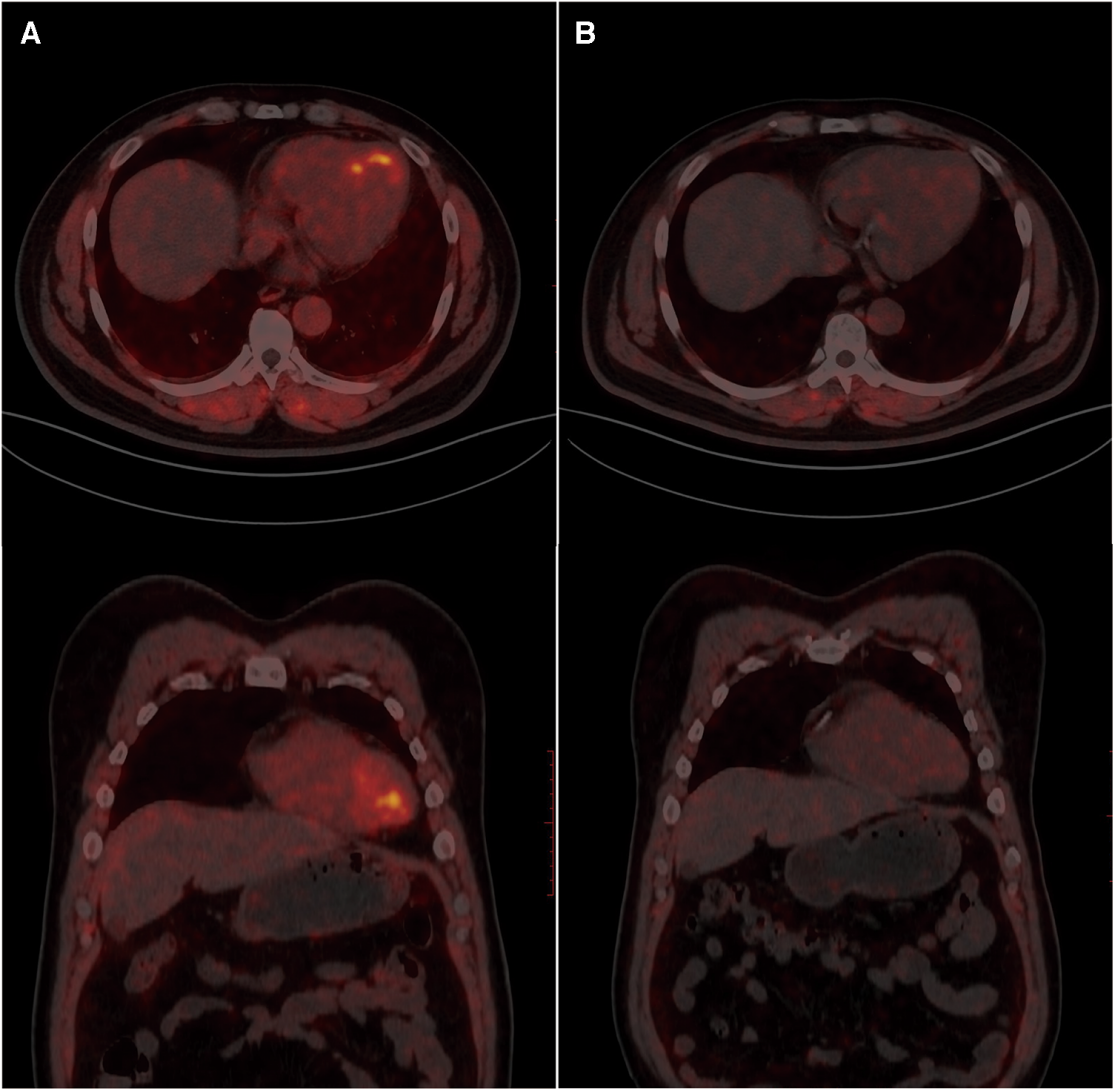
^68^Ga-FAPI-04 PET/CT myocardial imaging. A. Examination results one day after postoperative PCI. B. Follow-up examination results after two months.
